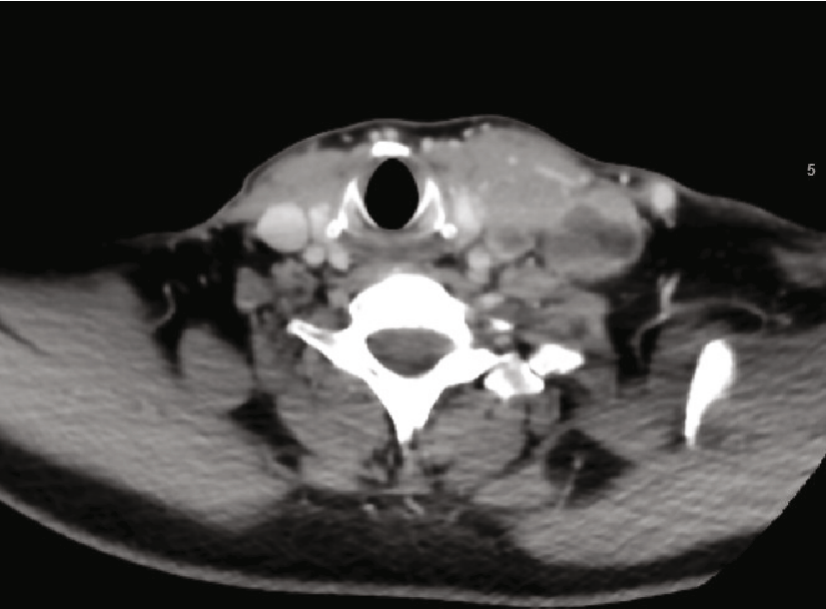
CT of the head and neck showing thyroid mass.
